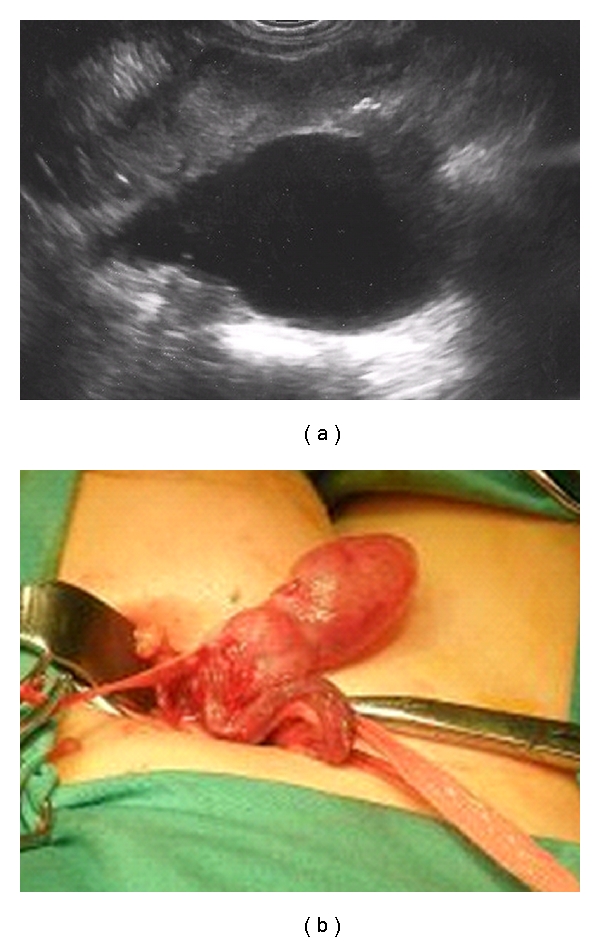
(a) Ultrasonic and (b) intraoperative images: the paratesticular mass, found to be an epididymal cyst was excised.
